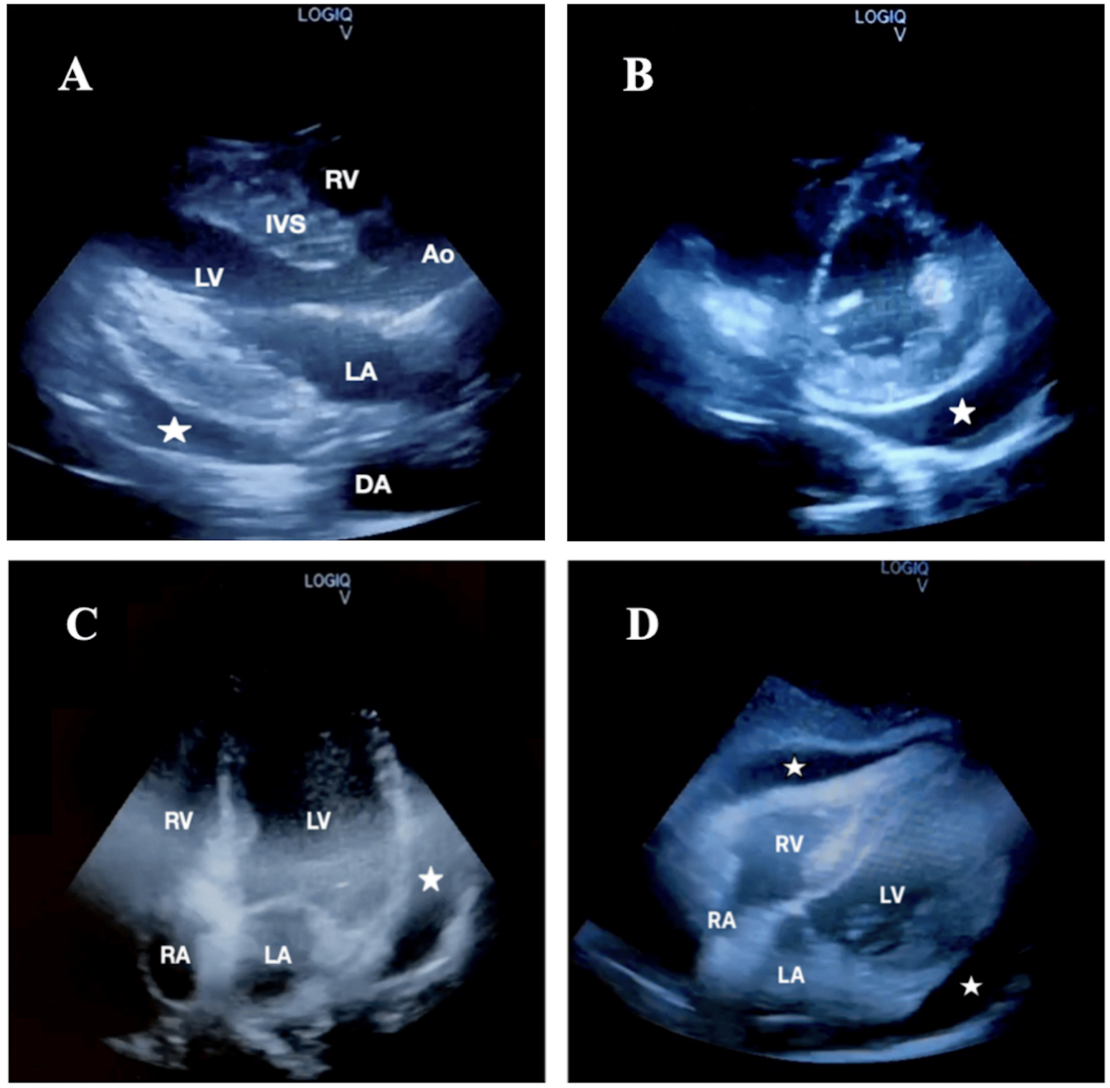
Standard echocardiographic views demonstrating pericardial effusion, indicated by the white star: (A) Parasternal long axis; (B) Parasternal short axis; (C) Apical four-chamber view; (D) Subcostal view Ao: aorta; DA: descending aorta; IVS: interventricular septum; LA: left atrium; LV: left ventricle; RA: right atrium; RV: right ventricle
